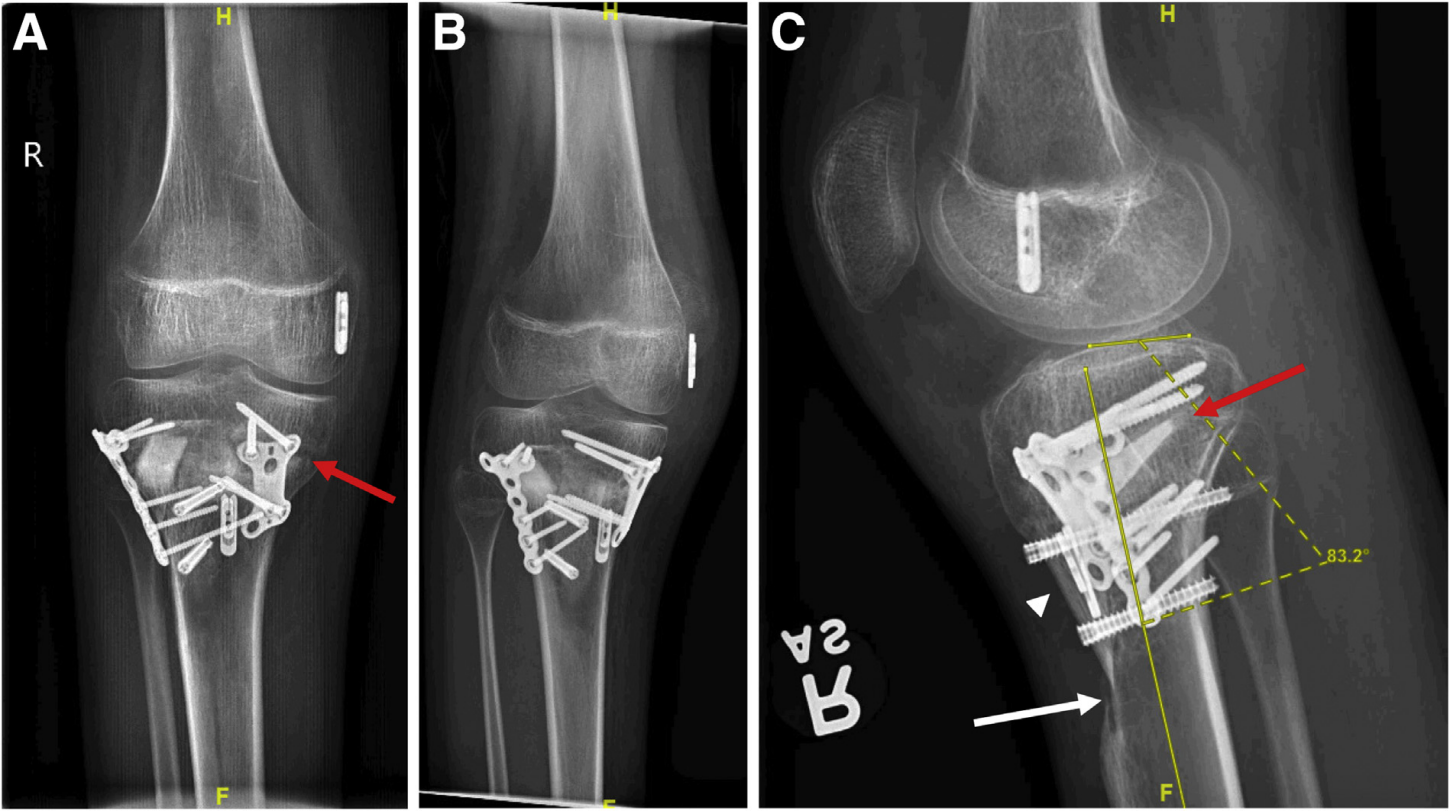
Six-week postoperative radiographs after combined high tibial osteotomy (HTO), tibial tubercle osteotomy (TTO), and posterior cruciate ligament reconstruction (PCL-R). Anteroposterior (A), oblique (B), and lateral (C) radiographs of the knee demonstrate a healed anterior opening wedge HTO and TTO with robust callus formation (red arrows) at the osteotomy site 6 weeks postoperatively. On the lateral radiograph (C), the tibial tubercle is proximal to its original location relative to the tibial diaphysis, as evidenced by the cortical defect (white arrowhead), in order to prevent patellar baja. The postoperative tibial slope is 7°, indicating that a 10° correction has been obtained.
